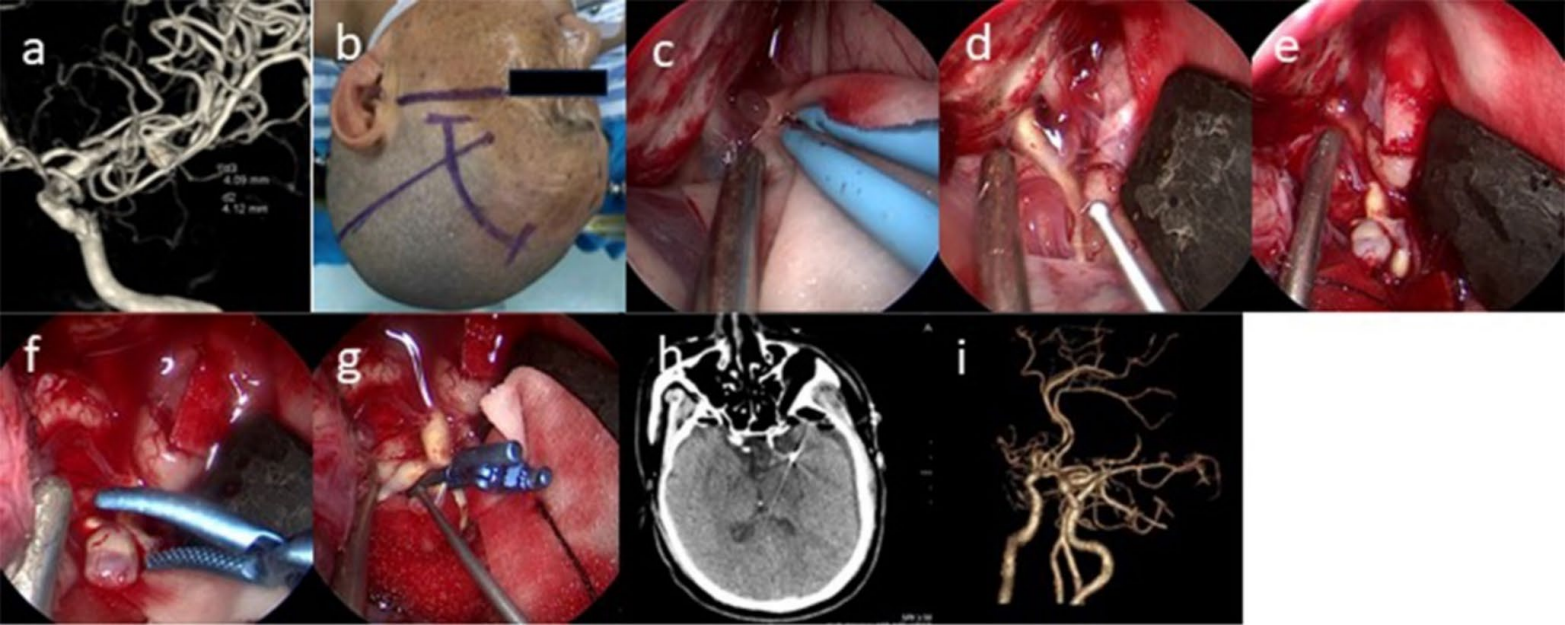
(a) Preoperative DSA confirmed the diagnosis of a left MCA aneurysm. (b) A PKA was selected for the left side. (c) The arachnoid membrane at the Sylvian fissure was opened, and CSF was released. (d-e) The aneurysm was unruptured, and intraoperative examination revealed a firm aneurysmal sac wall, which did not necessitate the use of a temporary clip. (f-g) The aneurysm was successfully clipped. (h) Postoperative CT scan showing no bleeding or ischemic changes. (i) Postoperative CTA scan showed that aneurysm was clipped completely and the parent artery and its branches were preserved
